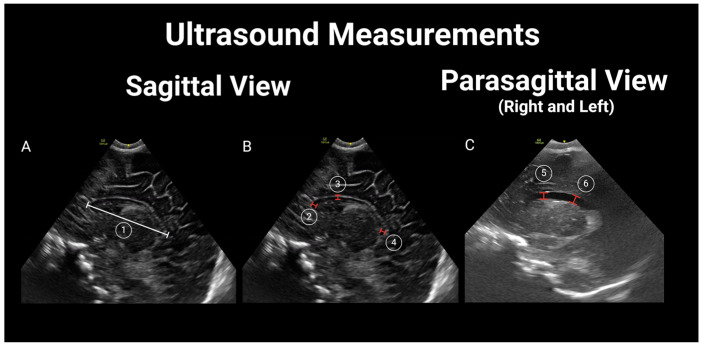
Ultrasound measurements in sagittal and parasagittal view. (A) 1. Corpus callosum length (CCL); (B) 2. Corpus callosum genu width (CCGW); 3. Corpus callosum body height (CCBH); 4. Corpus callosum splenium width (CCSW); (C) 5. Vertical height (VH); 6. Ventricle midbody height (VMH).
